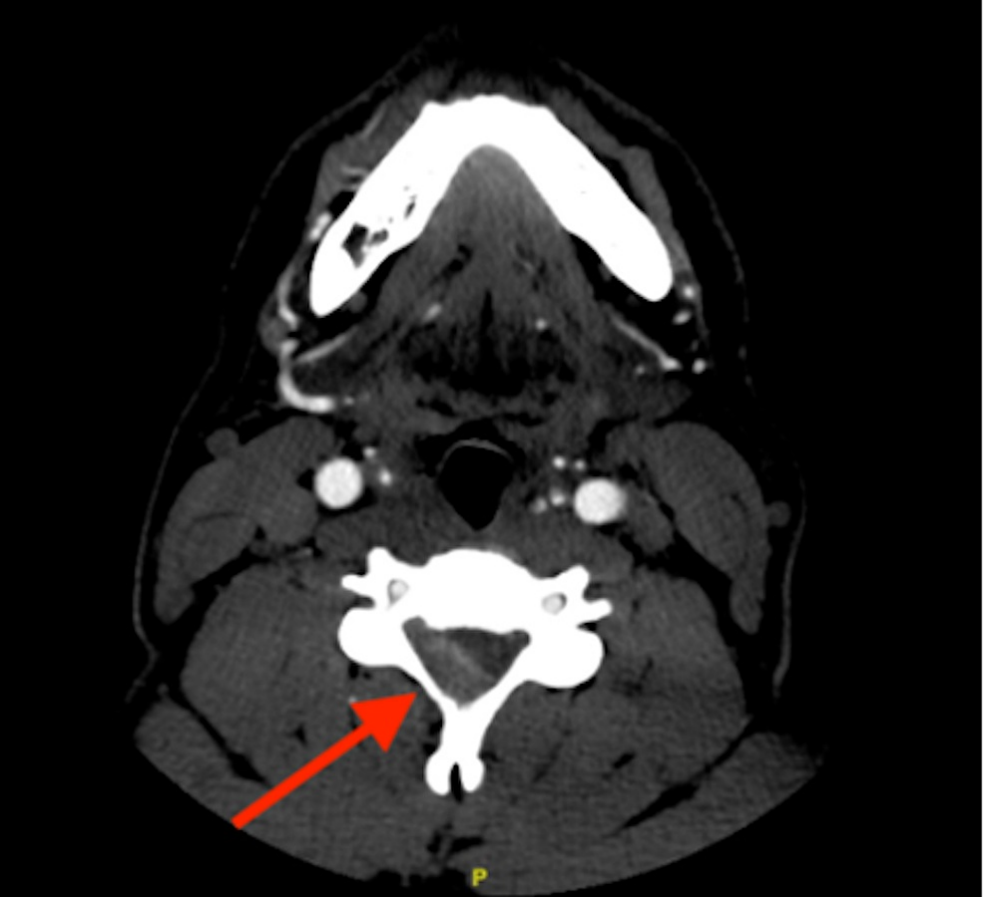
Axial CT with angiography image at the C3 level showing a right-sided epidural hematoma with an associated sac and cord compression in a left anterolateral direction
